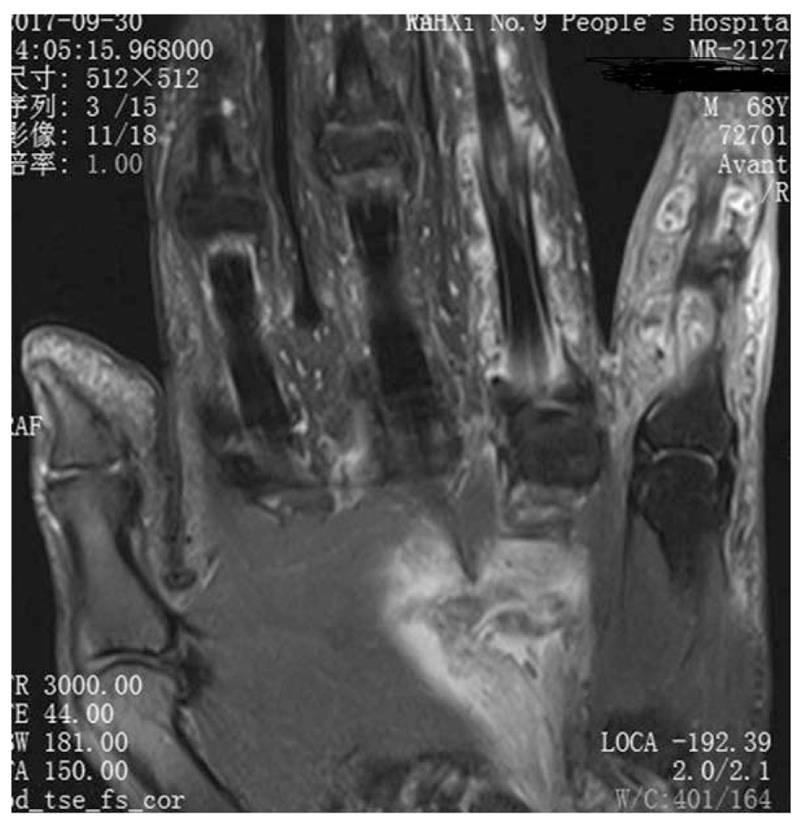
High signal in area of little finger and palm in T2-weighted sequences of MRI. MRI = magnetic resonance imaging.
